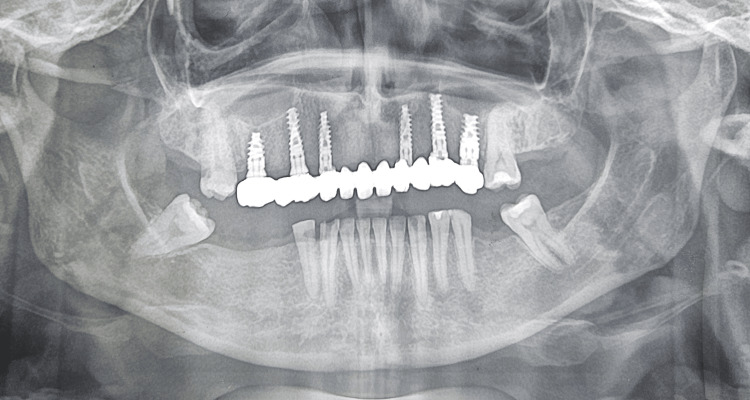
Postoperative orthopantomogram (OPG) Postoperative OPG evaluating the final positioning of the implants and prosthesis.
